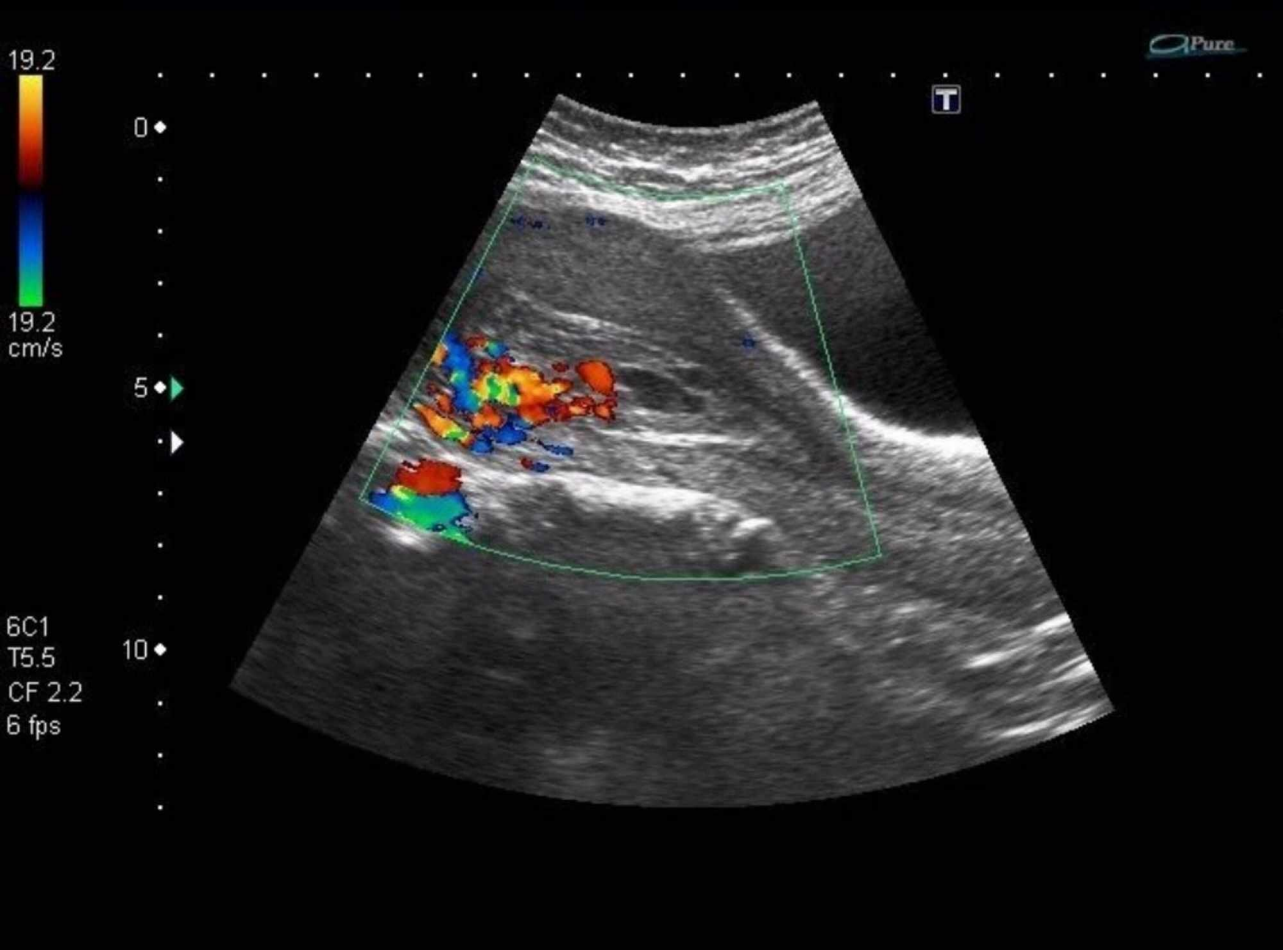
Doppler ultrasound showing hypervascularity in the endometrium
